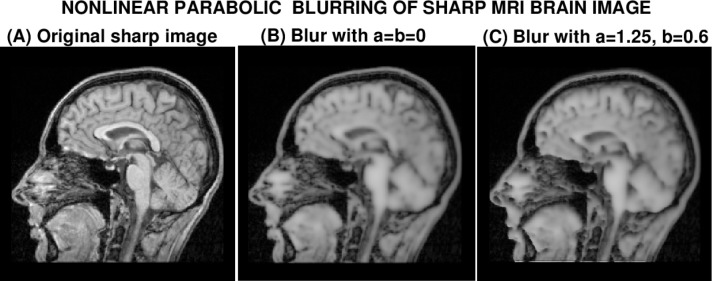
Nonlinear parabolic blurring of sharp MRI brain image *g*(*x*, *y*), by using it as initial values in Eq. (11) with two different sets of values for the constants *a*,*b*.
